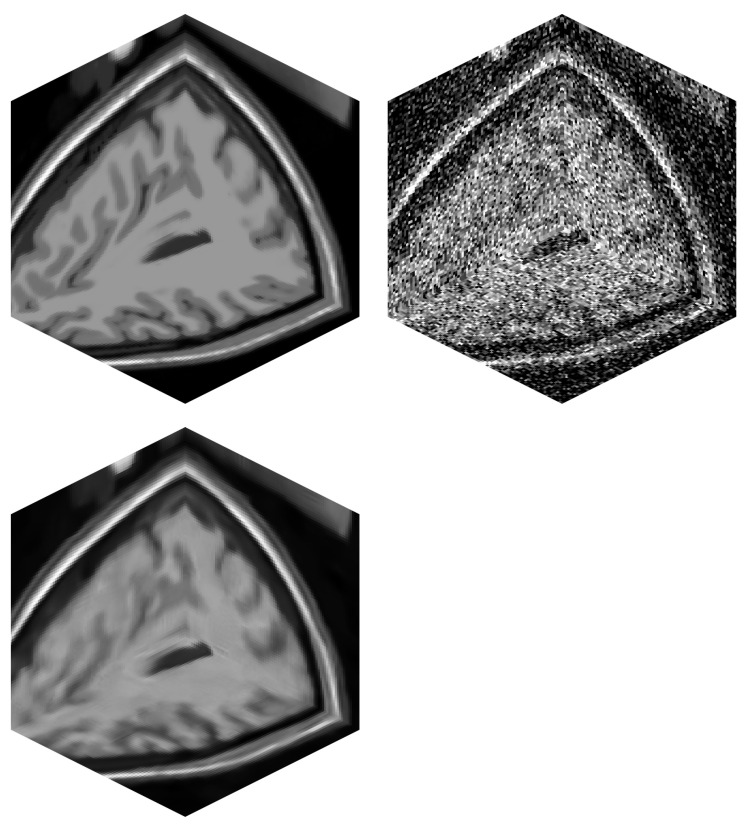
MRI volume of a brain (BrainWeb). (top left)—Original noise-less data. (top right)—Original data corrupted by 25% Gaussian noise. (bottom left)—Filtered image data by BM4D.
